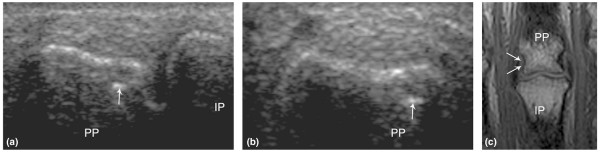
(a) Bone cortex defect (arrows) on ultrasonography in a 63-year-old healthy control person on the radial side of the 3rd proximal interphalangeal joint in a longitudinal view. (b) The corresponding transverse view. (c) The coronal T1-weighted magnetic resonance image without contrast administration reveals no erosion-like changes in the same person at the corresponding site (arrows). IP, intermediate phalanx; PP, proximal phalanx.
